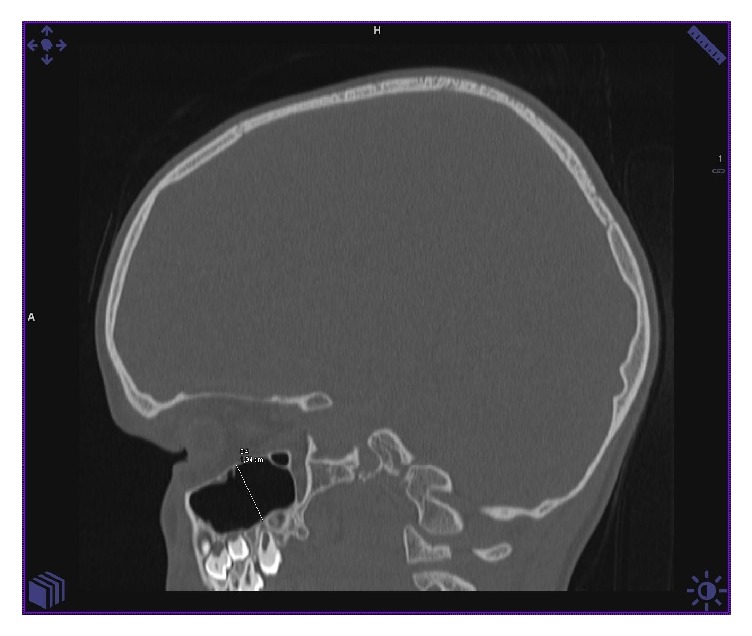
An example of MSH measurement on the CT image.
